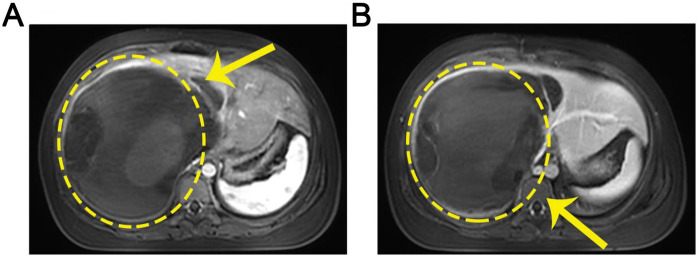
Preoperative MRI scans of the liver tumor. (A) Arterial phase. (B) Venous phase. The tumor is demarcated by yellow dotted circles in both T1-weighted images.
